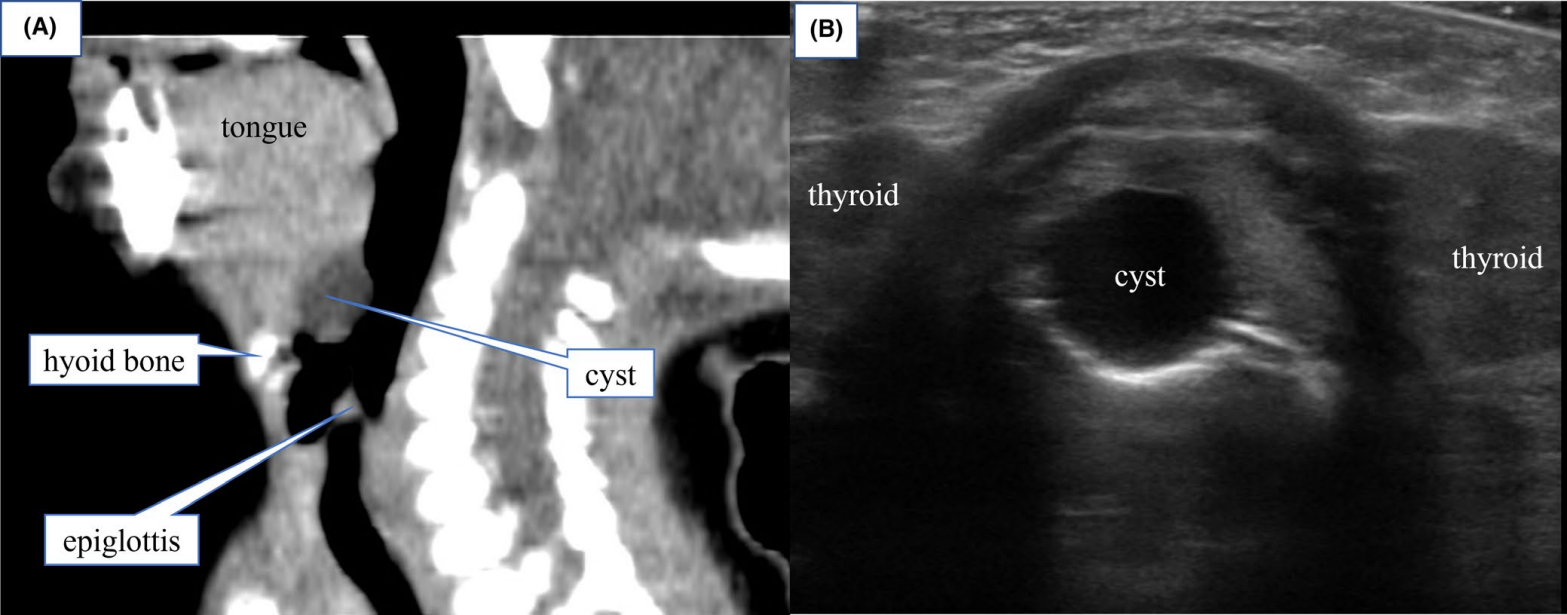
Computed tomography (CT) and cervical echography performed before laryngeal tumor resection. (A) Sagittal section of cervical CT showed a low‐density area about 1 cm in size at the tongue base. (B) Cervical echography revealed a cyst as a uniform hypoechoic region with a smooth margin
